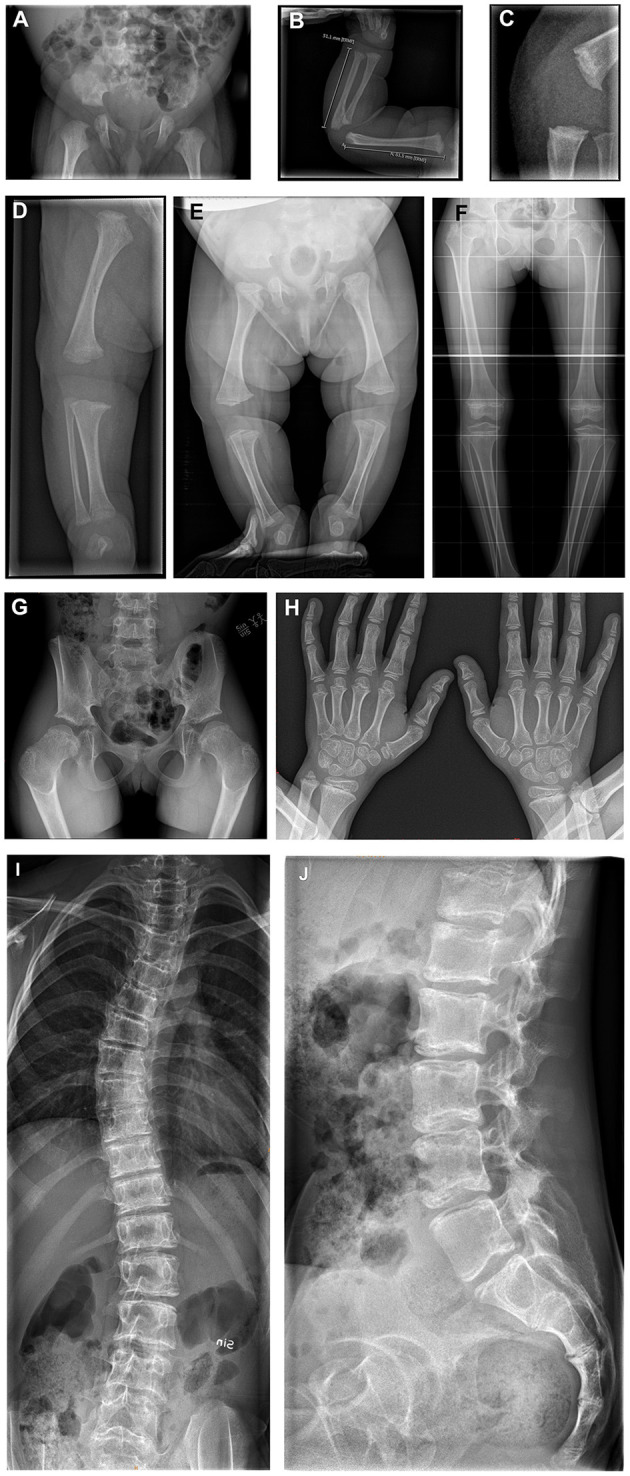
(A) X-rays of the skeleton of patients 3, 4, and 6. (A–D) Patient 3 at age 4 days; born with multiple congenital abnormalities of the bones. (A) The pelvis shows flat acetabula and short femoral necks. (B) The right arm demonstrates metaphyseal widening and irregularity. (C) The knee in the lateral view demonstrates metaphyseal irregularity in detail. (D) The image of the right leg demonstrates irregularly widened metaphyses at the distal femur and proximal and distal tibia, with a dysplastic knee joint and varus deformity. (E) Patient 4 at age 4 months; total legs, demonstrating flat acetubular roofs, wide femoral head metaphyses with short femoral necks. There is slight bowing and varus in the knees. The metaphyses around the knee show widening and irregularity. (F–H) Patient 6 at age 7 years. (F,G) Total legs and pelvic showing small iliac wings, coxa vara with small femoral heads and necks. This is shown in detail in image (G). The metaphyses around the knee demonstrate the typical striated sclerosis. The knee joints are dysplastic, and there is a varus deformity. (H) Both hands with irregularly widened and sclerotic metaphyses in the distal radius and ulna. Irregular metaphyses of the phalanges. (I,J) Patient 6 at age 12 years; total spine with scoliosis. The vertebral plates seem to have a double layer, with abnormal sclerosis of the plates. Lateral view shows the sclerosing of the vertebral plates from dorsal to ventral, resulting in a double contour.
